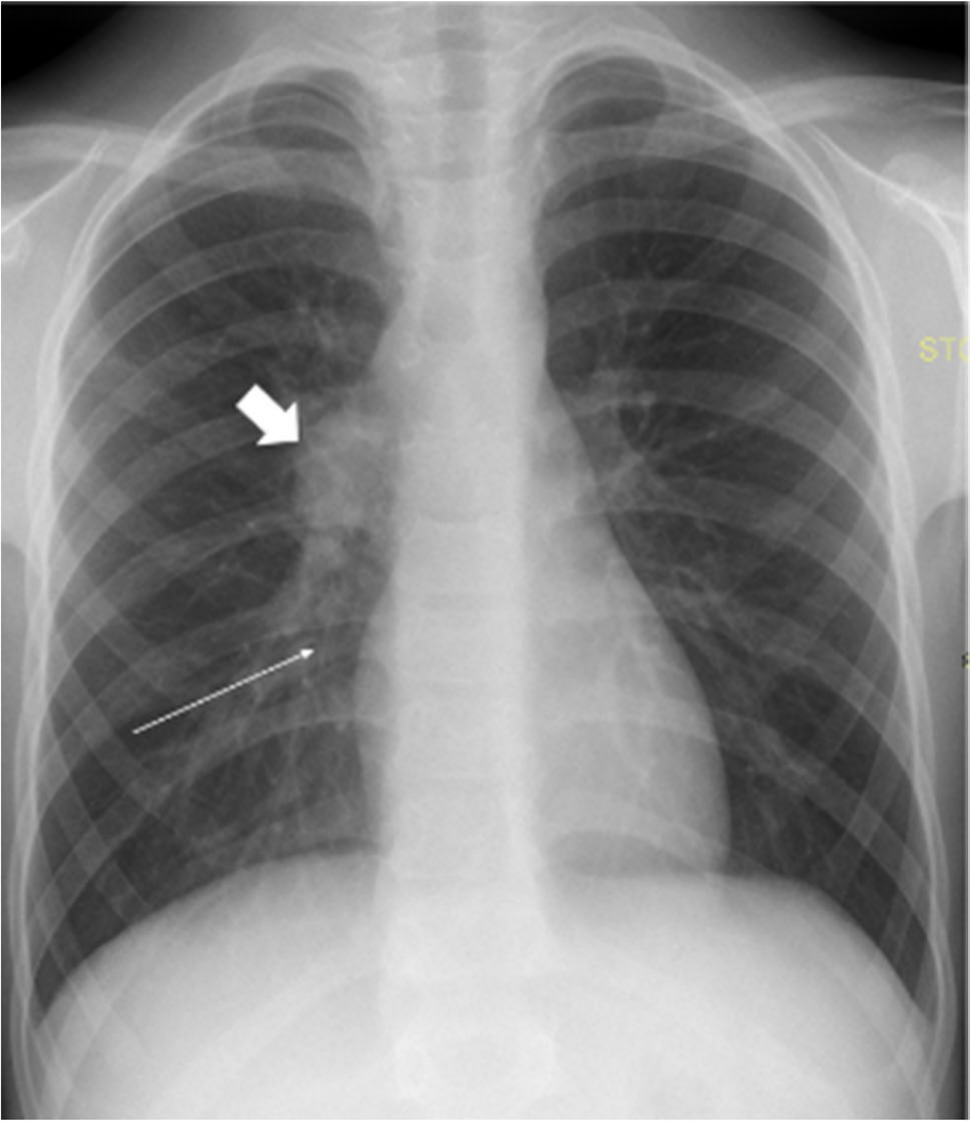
A posteroanterior chest radiograph in a 10-year-old boy with fever, cough, and malaise shows right hilar lymphadenopathy (*thick arrow*) and slight opacification within the right lower lung lobe (*thin arrow*). Ultrasonography (not shown) showed a small uncomplicated pleural effusion. Microbiological investigations confirmed tularemia
